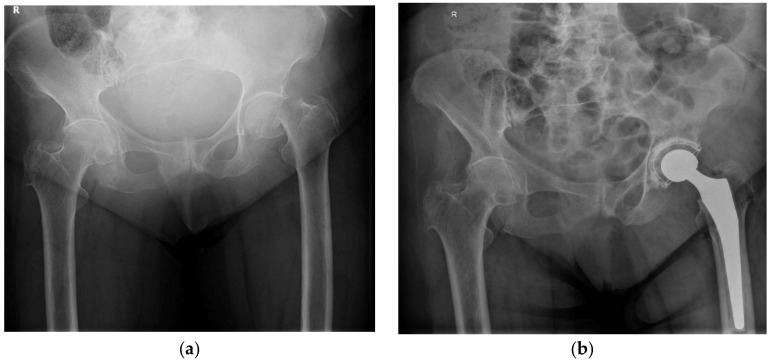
Radiographic evaluation of the left hip. (a) admission anteroposterior radiograph showing a displaced intracapsular femoral neck fracture (Garden IV); (b) postoperative radiograph after cemented total hip arthroplasty.
